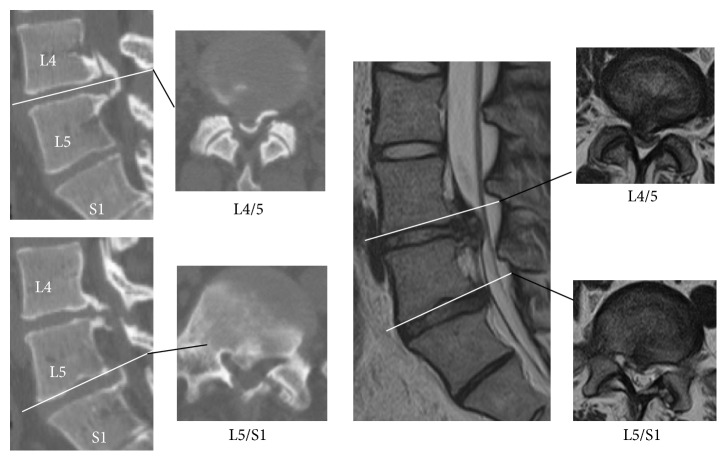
Computed tomography (CT) showed a huge ossification on the dorsal of annulus fibrosus of intervertebral disc at L4/5 at the age of 23 years. Ossification at L4/5 was larger than that at L5/S1. The ossification was consecutive with the posterior end plate at L4/5 and L5/S1. CT did not show an abnormal ossification of annulus fibrosus or posterior longitudinal ligament at the other levels. MRI revealed severe stenosis at L4/5 and the lesion of the posterior endplate lesion still remained at L5/S1.
